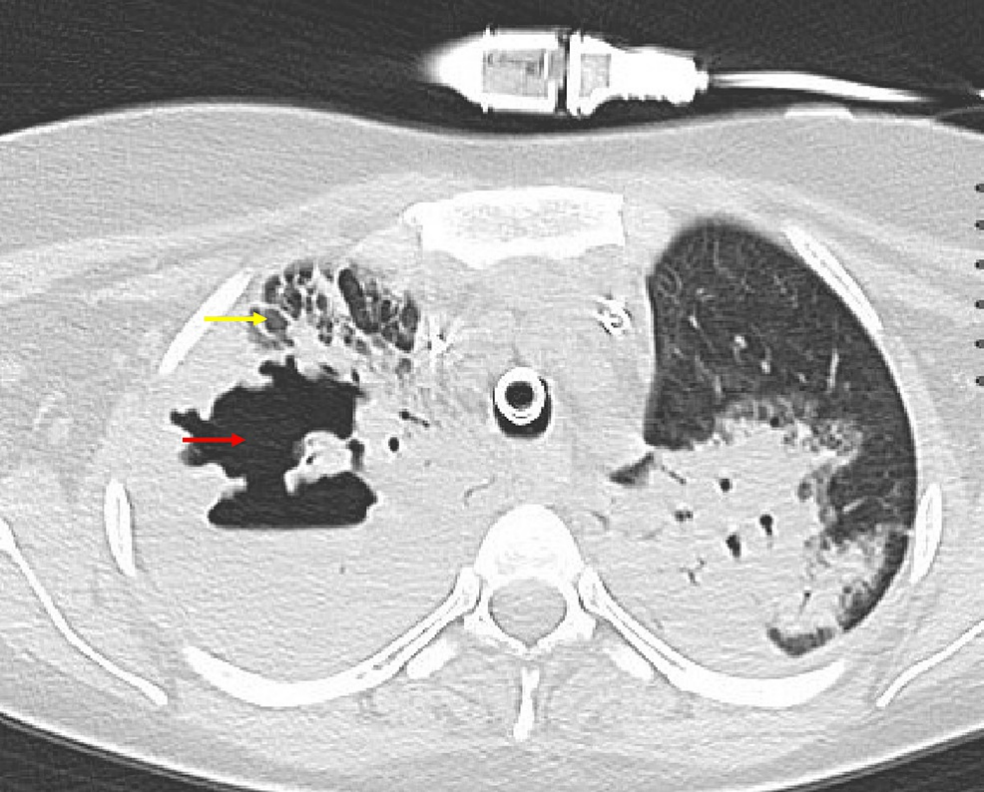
Non-contrast Computed Tomography of the Chest The scan demonstrates complete right upper lobe consolidation (yellow arrow) and a necrotizing cavitary lesion (red arrow).
